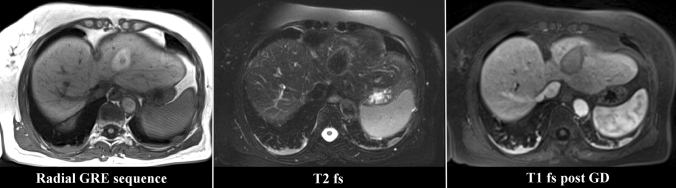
Visibility of the ablation zone indicated by parenchymal hemorrhage in the radially acquired free-breathing 3D stack-of-stars gradient echo sequence (Radial GRE). T2-weighted fat saturated (T2 fs) and T1-weighted fat saturated after gadolinium-based contrast (T1 fs post GD) are shown for comparison
